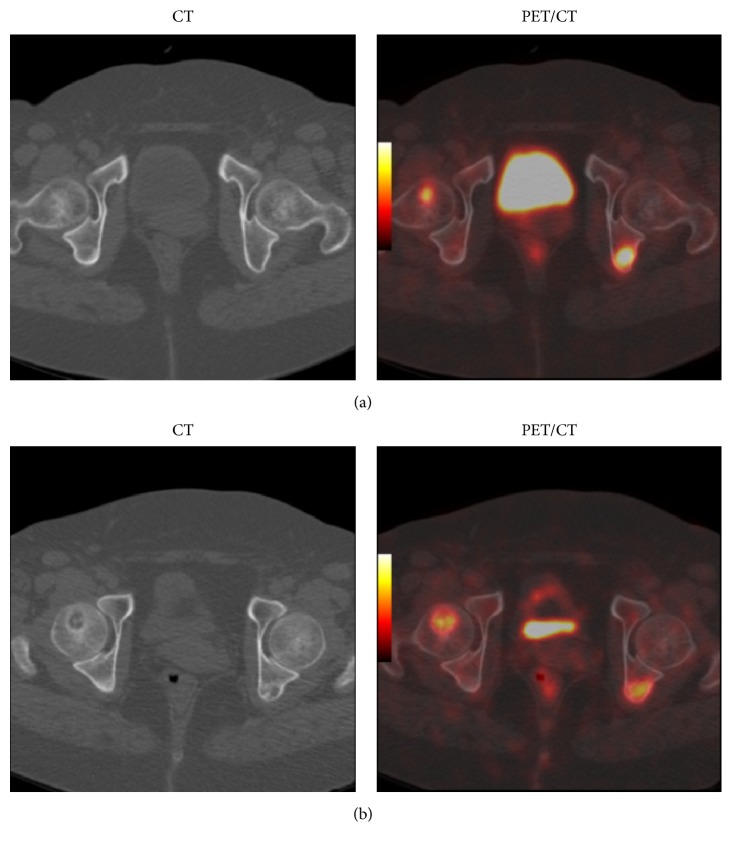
Breast cancer patient (51 years old) evaluated by F18-FDG-PET/CT showing two bone marrow metastatic lesions on baseline scan (a) which progressed to FDG-positive osteolytic metastatic bone lesions on 3 mo follow-up scan (b).
